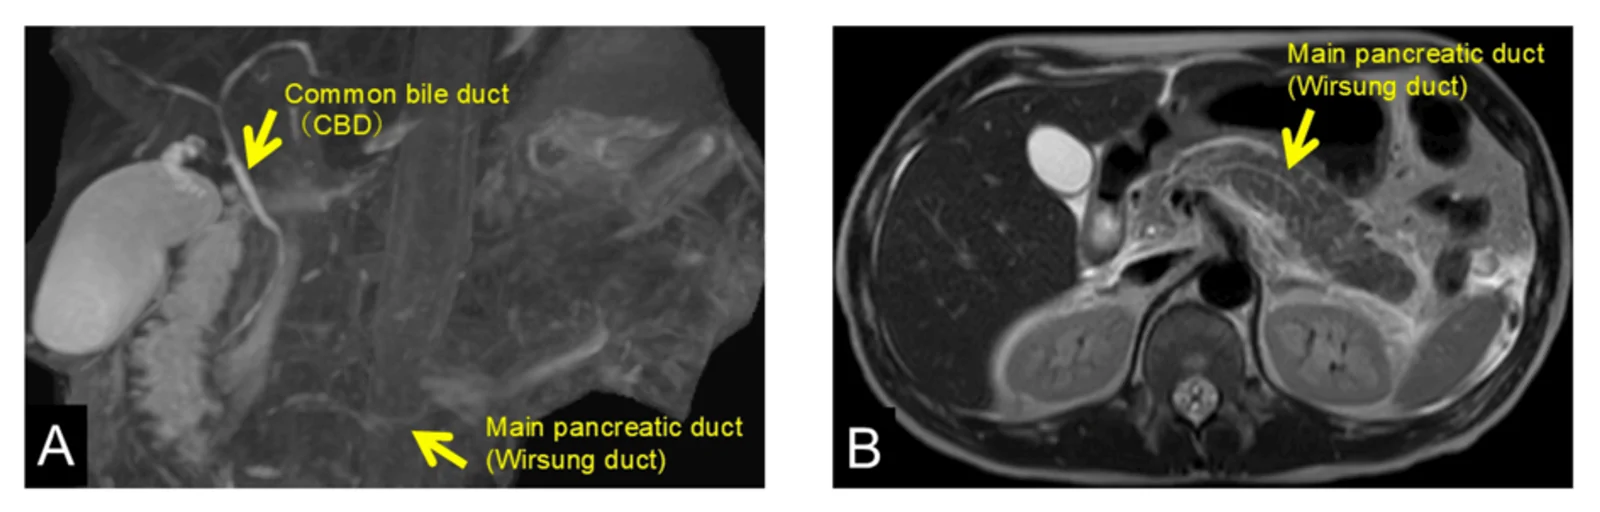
MRCP findings during the episode of acute pancreatitis in 2023. (A) Three-dimensional maximum intensity projection image and (B) axial T2-weighted image demonstrating no significant pancreatic duct dilatation or structural abnormalities. Yellow arrows indicate the main pancreatic duct (Wirsung duct) and the common bile duct (CBD). MRCP, magnetic resonance cholangiopancreatography.
